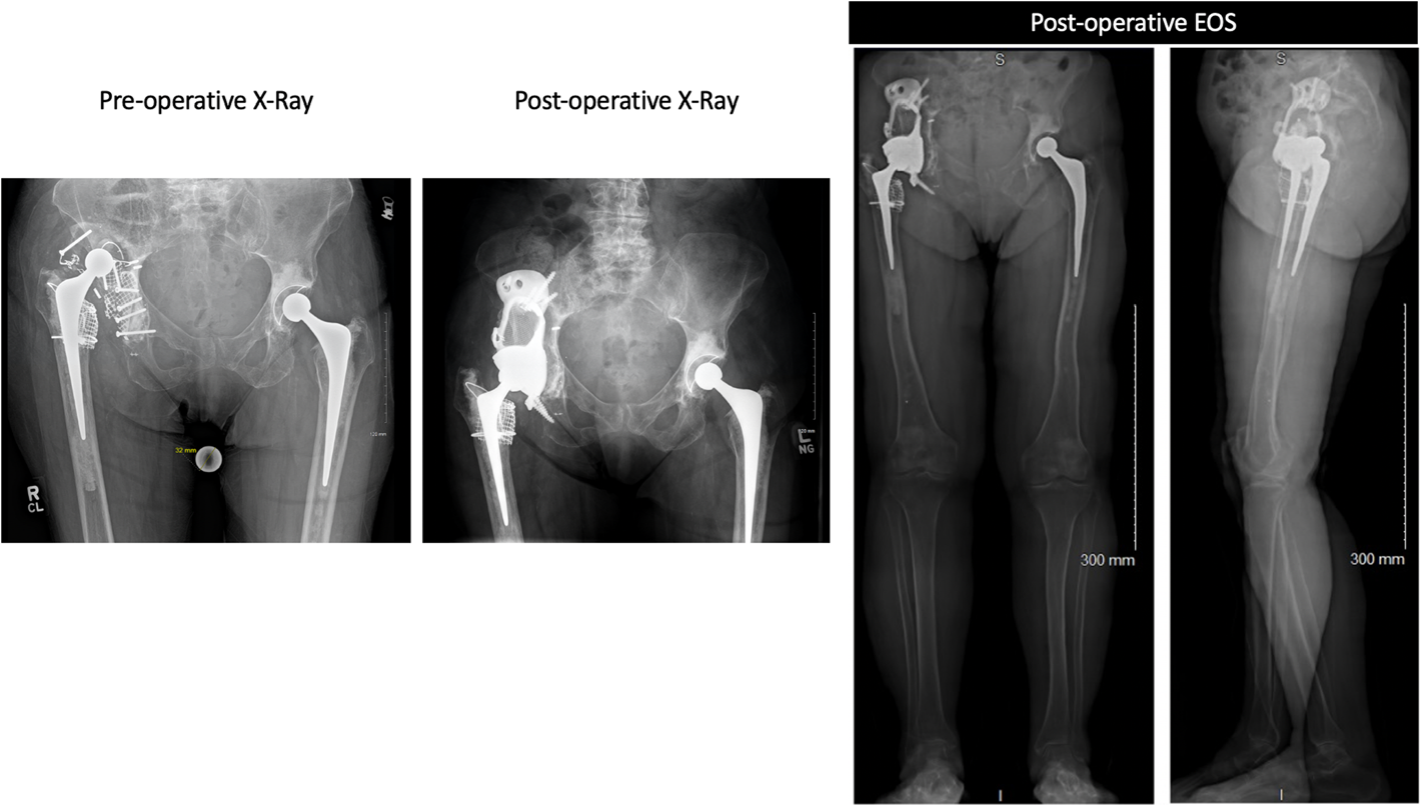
Case example. Pre-operative AP X-Ray (left image) showing major migration of the right hip, discrepancy between right and left VFO was 5 cm. Post-operative pelvic AP X-Ray (middle images) shows good restoration of centre of rotation, VFO discrepancy was 8 mm. Functional LLD measured on post-opeartive EOS imaging (right image) was 18 mm. Knee flexion can be observed on the lateral EOS image (left knee flexion =18°, right flexion =3°), difference in femur length = 2.5 cm
